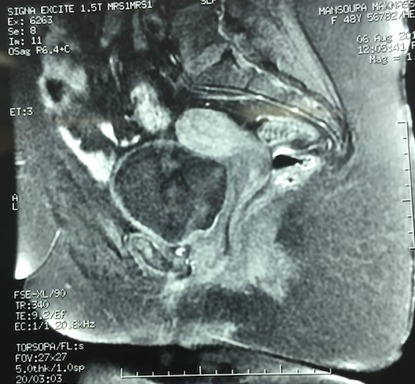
Pelvic MRI (T1): vaginal narrowing
